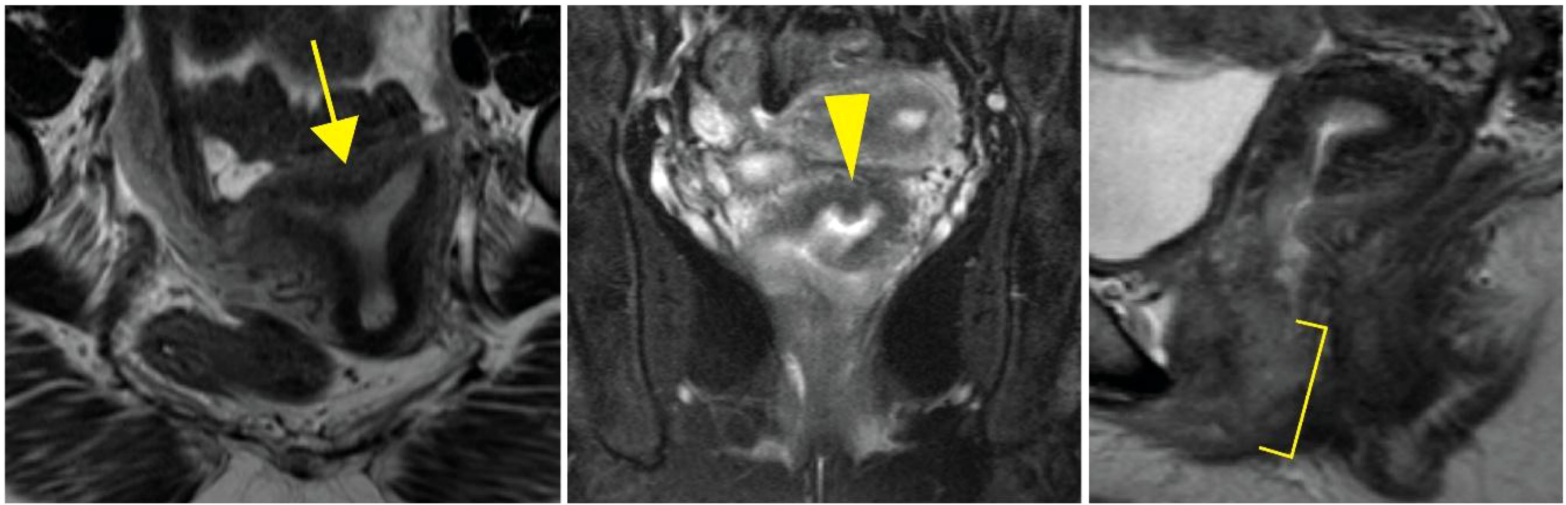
Arcuate uterus in an individual harboring *WT1* c.1338A>C. Axial (left), coronal (middle), and sagittal (right) MRI images demonstrating atypical uterine morphology. The arrow indicates arcuate uterine morphology. The arrowhead indicates a lack of clear uterine septation. The bracket highlights atypical vaginal morphology without a clear vaginal septum.
